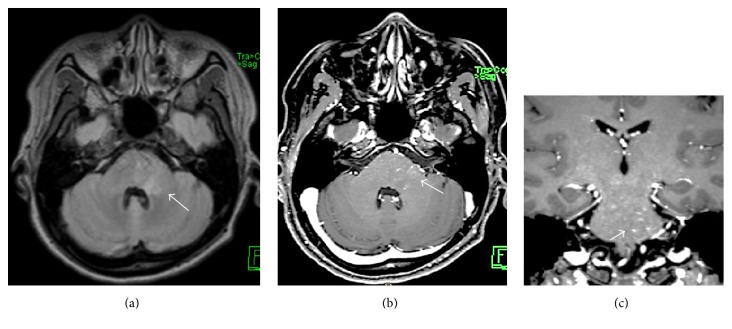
(a) Axial T2/FLAIR and (b) axial and sagittal (c) T1 weighted MRI after IV gadolinium based contrast medium injection, showing ill-defined faint bright intensity in the posterior pons and left brachium pontis (arrow in (a)) and characteristic punctate and linear enhancement (arrow in (b) and (c)).
